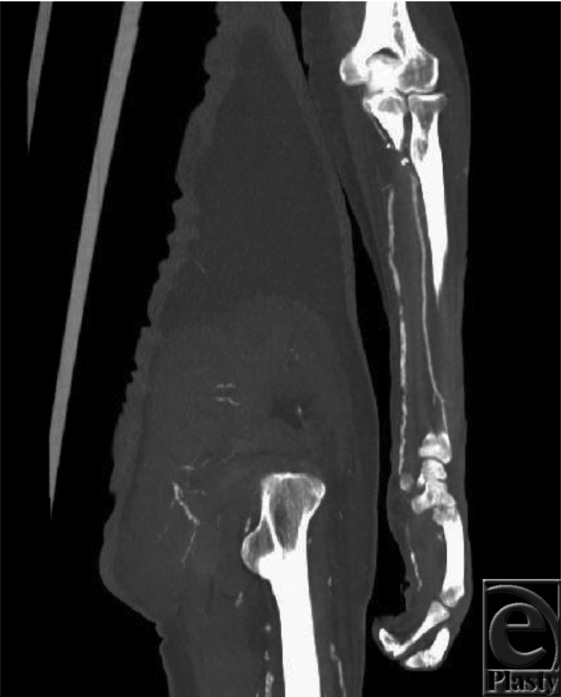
(a) Doppler ultrasonography report showing poor left hand perfusion. (b) Computed tomography angiography of left upper extremity demonstrating calcified arteries and diminished flow around the level of the wrist.
